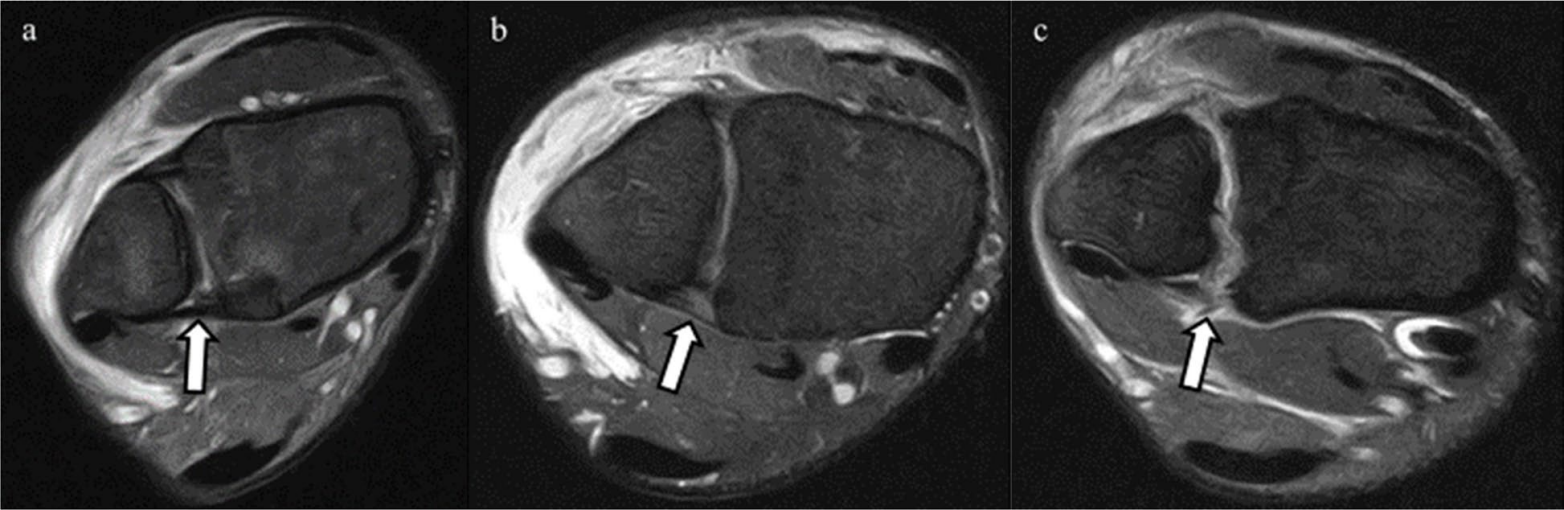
Posterior inferior tibiofibular ligament (PITFL) in oblique axial proton-density fat saturation MRI. The arrows in the figures indicate PITFL. a The intact PITFL. b Partial tear of the PITFL, with hyperintensity of the ligament. c Complete tear of the PITFL, showing complete discontinuity
